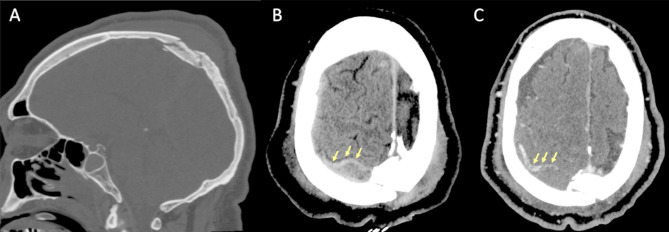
CT scan of a patient in the early sixth decade of life with a depressed skull fracture of the parietal bone (A), with findings consistent with thrombosis of a cortical vein. Non-contrast CT scan (B) shows a linear hyperdensity at the level of the right post-central sulcus (yellow arrows) along the presumed anatomical course of a cortical vein, adjacent to the fracture. Follow-up CTV at 48 h (C), shows absent/irregular opacification along the course of a right parietal cortical vein (arrows), corresponding to the non-contrast finding, consistent with cortical vein thrombosis. The superior sagittal sinus (not shown) was patent
